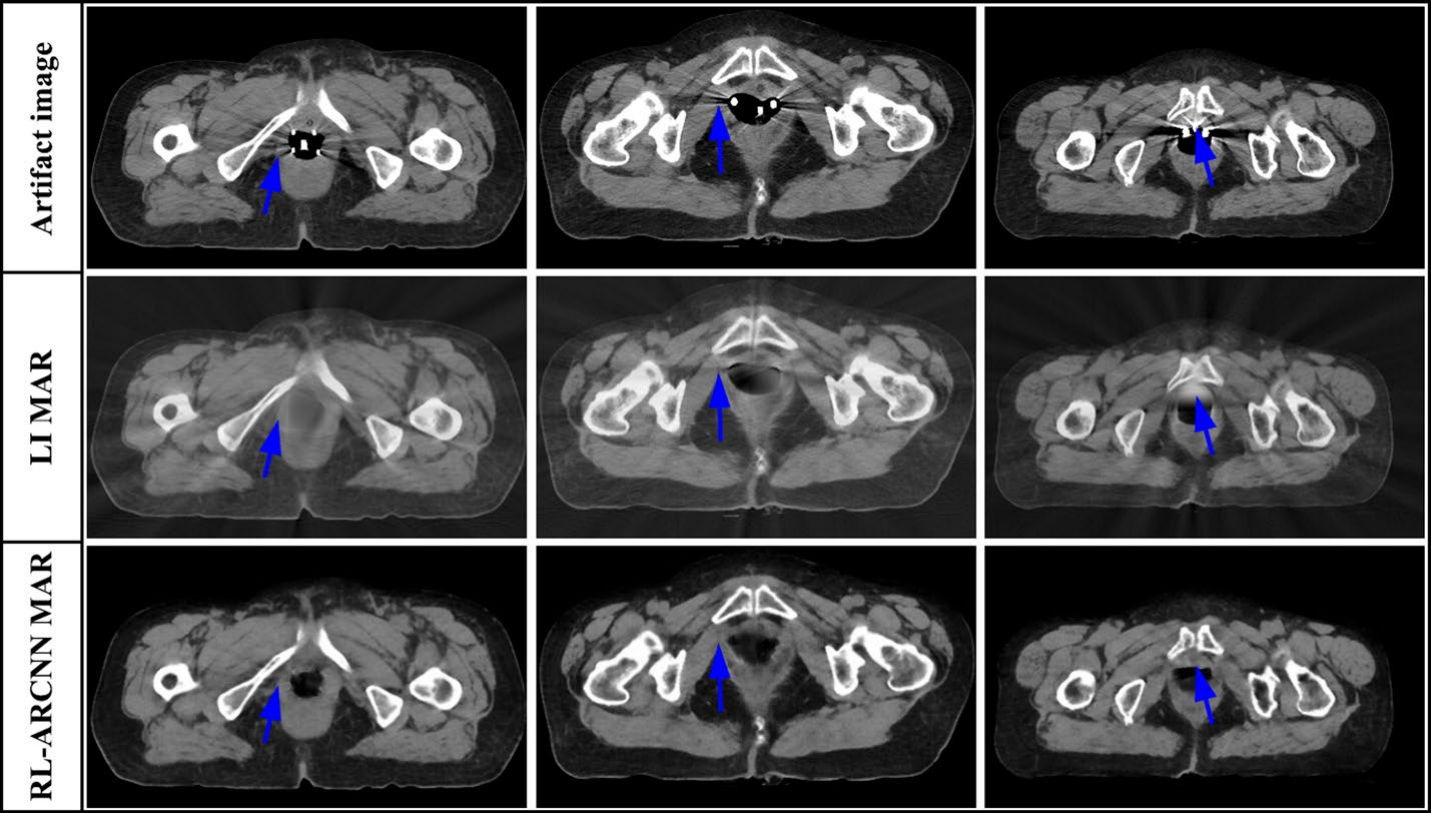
Visual comparison between the MAR results of RL-ARCNN and LI method. The first row is CT images with metal artifacts, and the second and third row are the metal artifacts reduction images by the LI and RL-ARCNN, respectively
